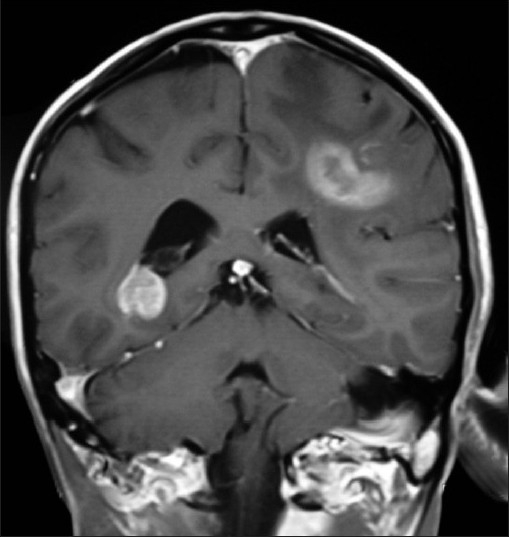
T1WI coronal MRI brain of an orchard worker showing multicentric glioma
